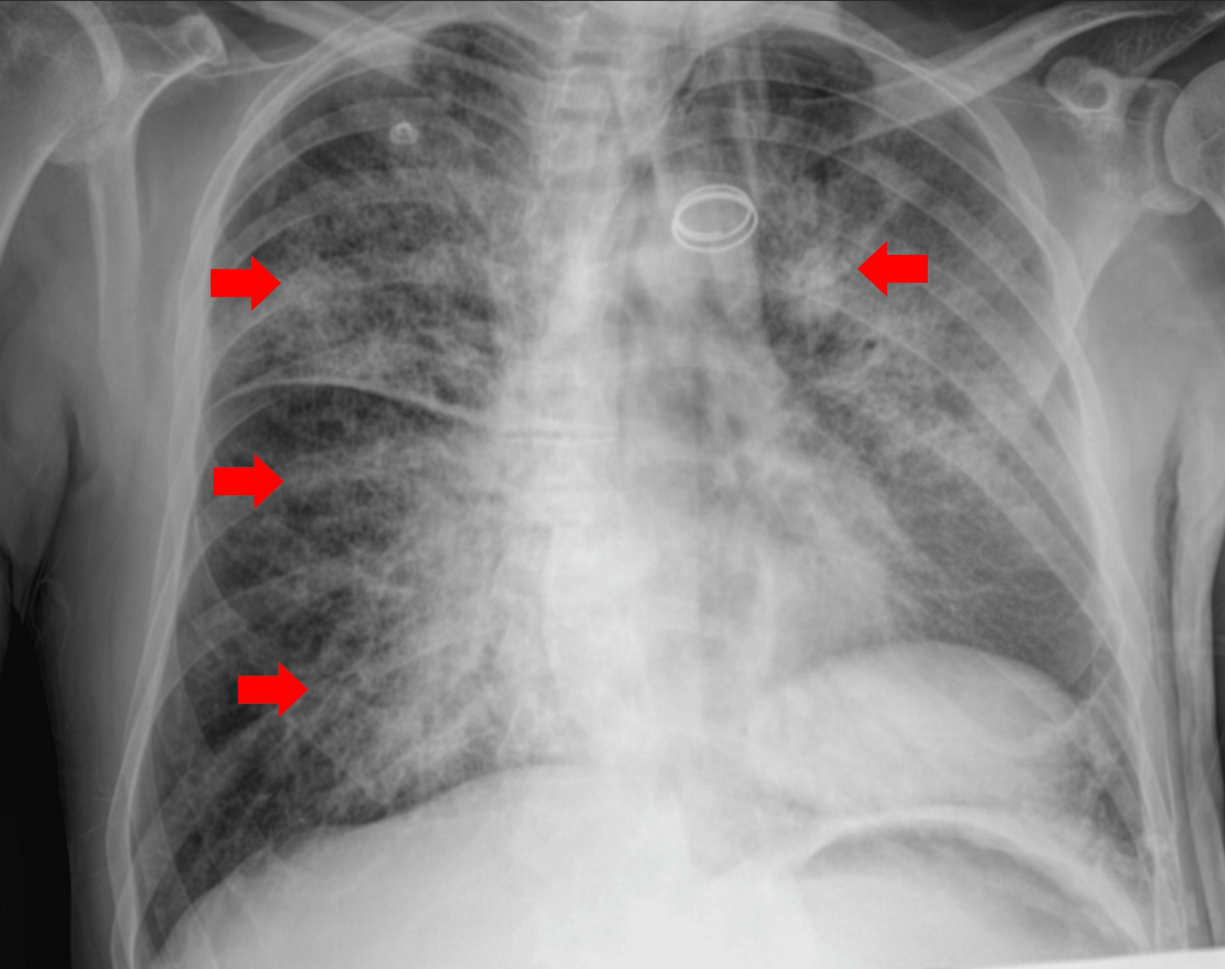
Chest X-ray showing a diffuse bilateral reticulonodular pattern (red arrows), consistent with the patient’s presentation of acute respiratory distress syndrome (ARDS).
